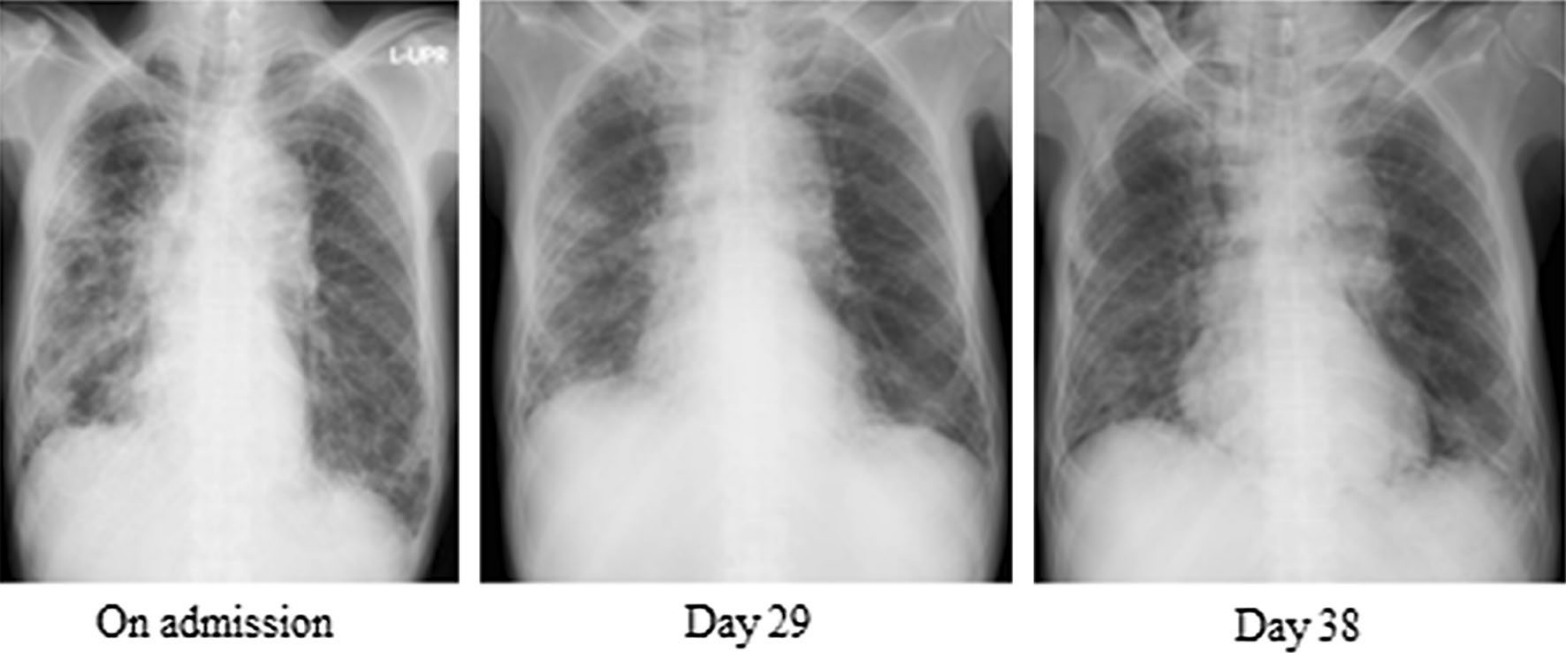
Chest radiography did not show apparent deterioration in association with the serum ferritin level
